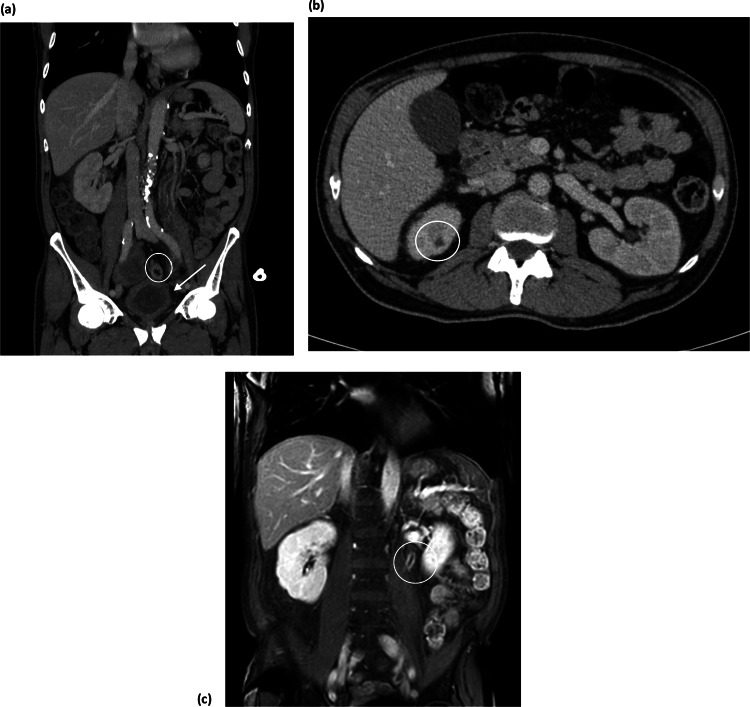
Abdominal imaging demonstrating extensive involvement of the urinary tract. a Coronal view of contrast-enhanced abdominal computed tomography: The circle indicates thickening of the left ureter. The arrow indicates diffuse bladder wall thickening. b Axial view of contrast-enhanced abdominal computed tomography: The circle shows a developing perirenal abscess. c Coronal view of contrast-enhanced T1-weighted sequence with subtraction in the venous phase on urinary tract MRI: The circle indicates diffuse ureteral thickening
